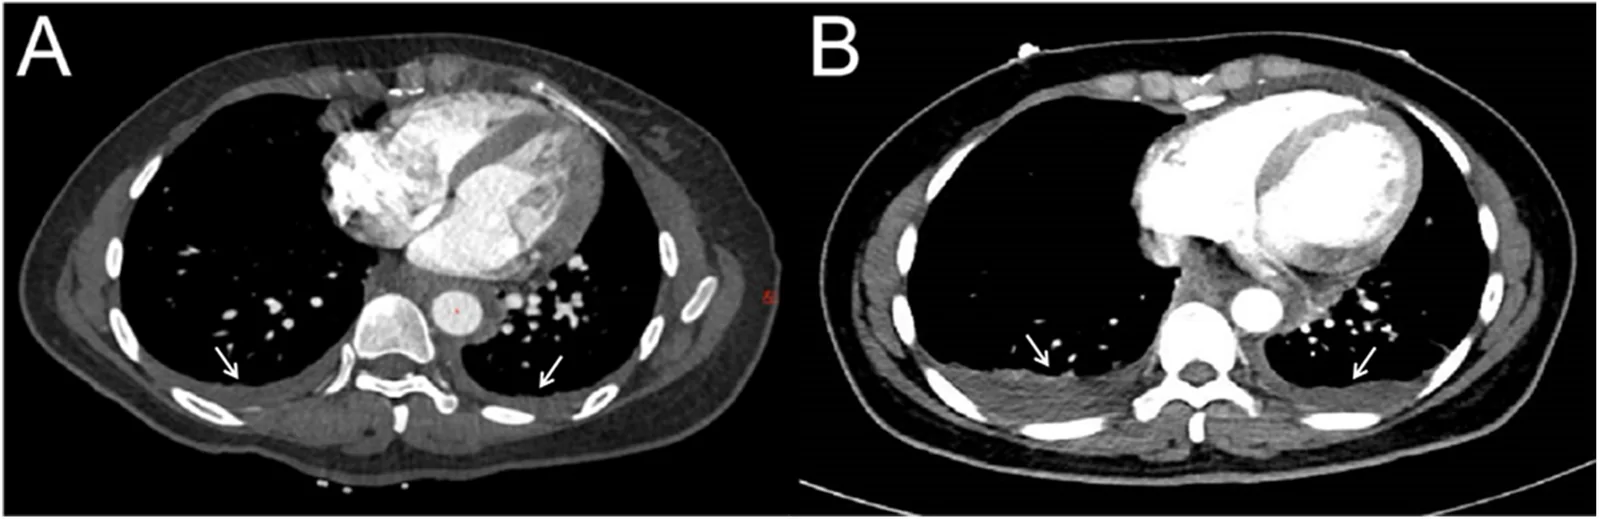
Axial computed tomography (CT) images demonstrating bilateral pleural effusions. (A) Initial CT performed on March 15, 2022, showing bilateral pleural effusions (white arrows). (B) Repeat CT performed 10 h later demonstrating a marked increase in bilateral pleural effusions (white arrows).
